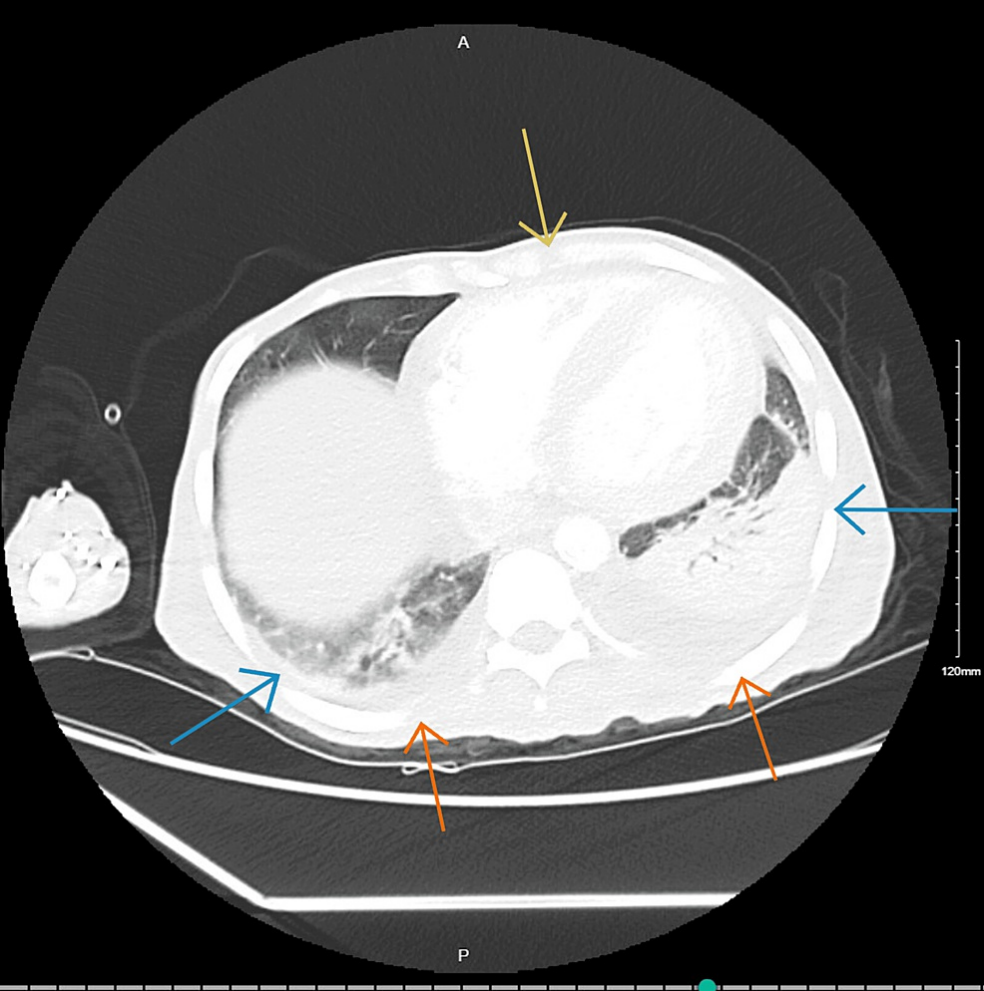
CT chest with contrast showing consolidations within lower lungs (blue arrows), cardiomegaly (yellow arrow), and bilateral pleural effusions(orange arrows).
